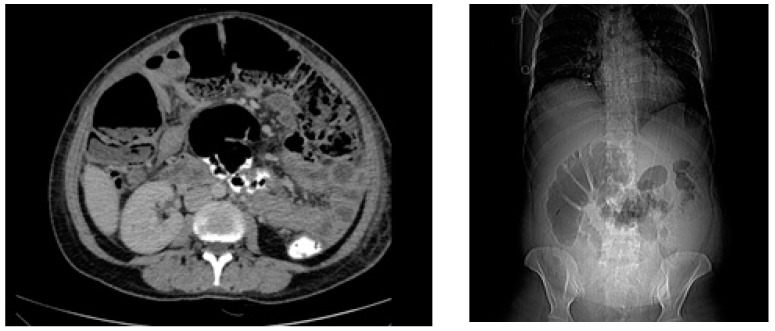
Abdominal CT at presentation indicating colonic dilatation with colonic wall thickening was consistent with ileus without obstructing mass or collection.
